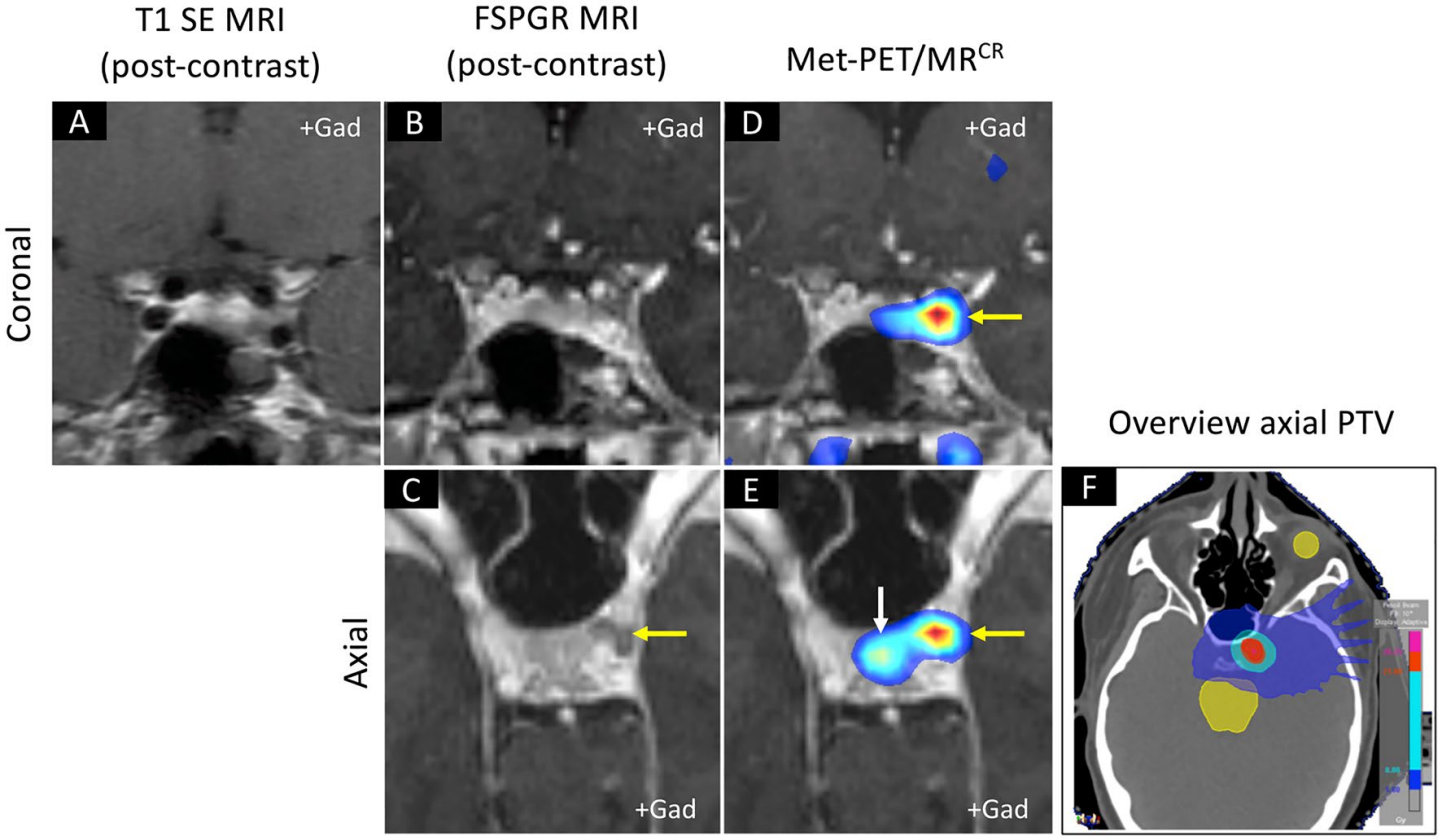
PET–guided stereotactic radiosurgery in case 10. A–B Post-contrast coronal T1 SE and FSPGR MRI demonstrate indeterminate appearances in a patient who had previously undergone transsphenoidal surgery for a left-sided microprolactinoma. C Axial FSPGR MRI shows possible recurrent tumor in the left cavernous sinus (yellow arrow). D–E Coronal and axial Met-PET/MR^CR^ confirm avid tracer uptake at the site of the suspected recurrence (yellow arrow); tracer uptake within the remaining normal gland is also seen (white arrow). F Treatment plan for PET-guided SRS. Three years later serum prolactin was near-normalized (1.4 × ULN). *FSPGR* fast spoiled gradient recalled echo, *Gad* gadolinium, *MRI* magnetic resonance imaging, *Met-PET/MR*^*CR*^
